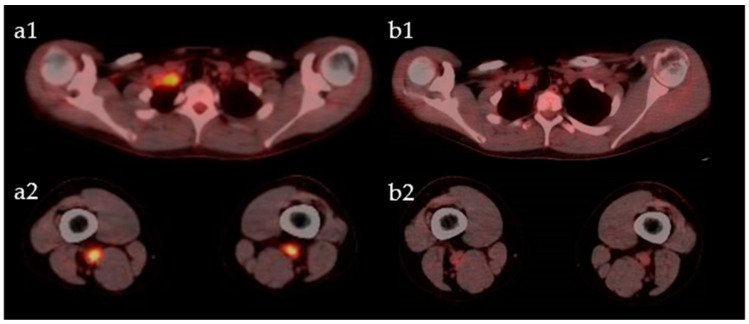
FDG-PET images. FDG accumulation in right subclavian artery (a1) and bilateral femoral artery (a2) before administration of tocilizumab. FDG accumulation in right subclavian artery (b1) and bilateral femoral artery (b2) a few months after discontinuation of tocilizumab. SUVs are improving, indicating that Takayasu’s arteritis is little activity.
